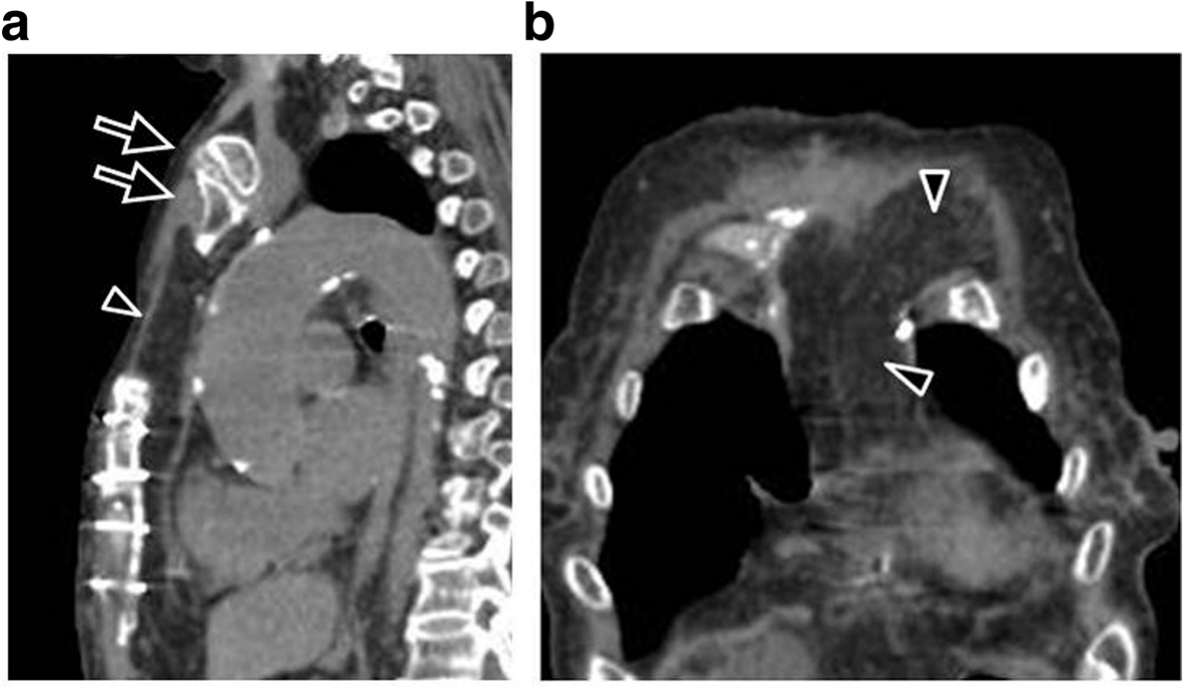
Contrast-enhanced computed tomography two months postoperatively. a Sagittal image. b Axial image. The manubrium of the sternum was intact (*arrows*) and a healthy omental flap covered the sternal defect (*arrowheads*). Postoperative computed tomography
